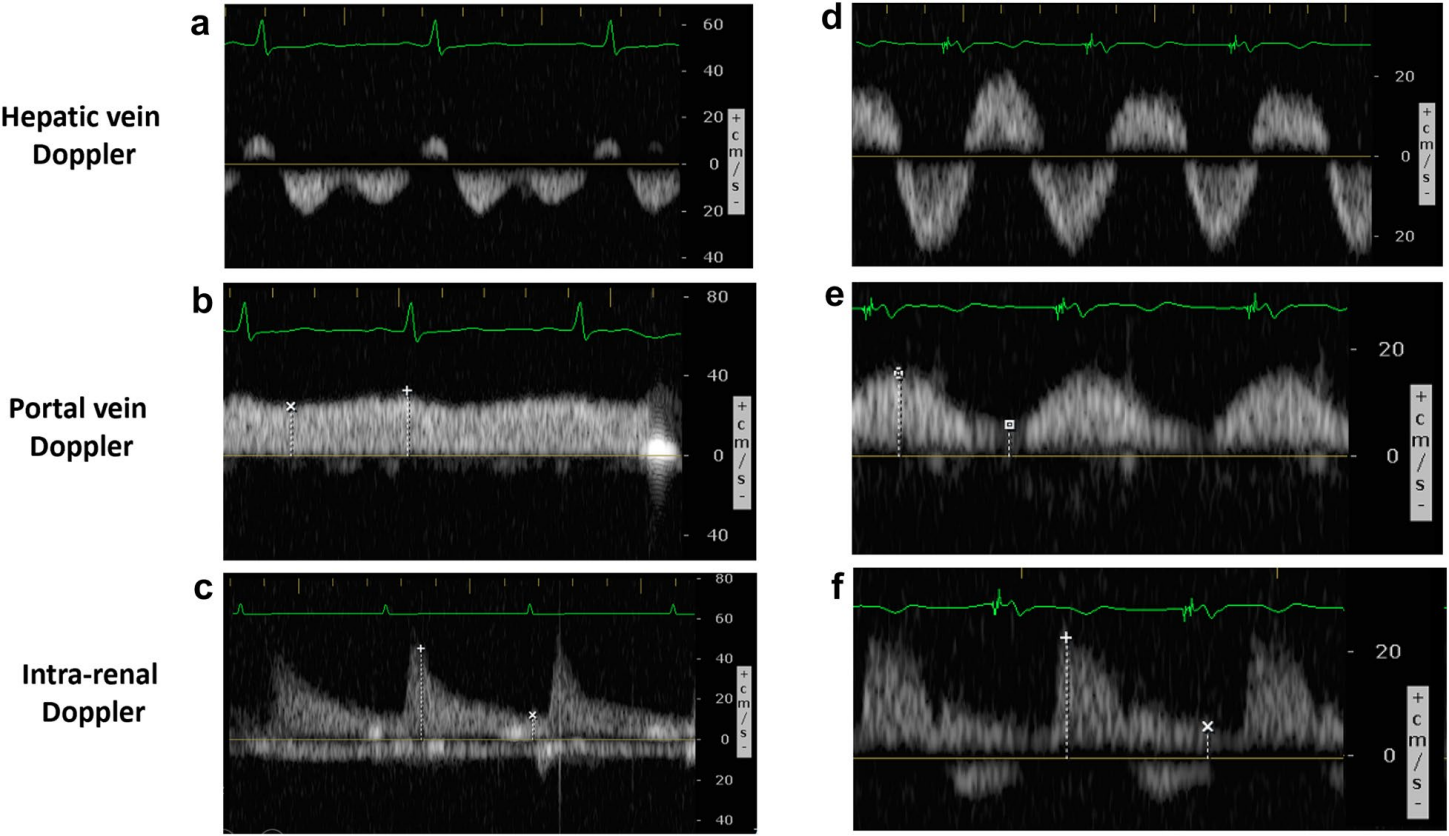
Example of VExUS C grading system assessment in cardiac surgery. Patient #1: A 55-year-old woman undergoing tricuspid valve repair aortic valve replacement and mitral valve replacement known with chronic kidney disease (baseline eGFR = 35 mL/min/1.73 m^2^) with left ventricular ejection fraction of 40% and a high risk of major complications (EuroSCORE II = 16.8%) presented the following ultrasound findings at ICU admission after surgery: a Normal hepatic triphasic pattern, b a non-pulsatile portal flow and c continuous intral-renal venous flow and an IVC diameter of > 2.1 cm (not shown) corresponding to Grade 1 of VExUS C grading system. The patient did not develop acute kidney injury, was extubated 2.2 h after ICU admission and was discharged from the ICU less than 24 h after surgery. Patient #2: A 70-year-old man undergoing mitral valve repair with a left ventricular ejection fraction of 50% and a moderate risk of major complication (EuroSCORE II = 1.54%) presented the following ultrasound findings at ICU admission after surgery complicated by right ventricular dysfunction after cardiopulmonary bypass: d Systolic reversal of the hepatic venous flow, e severe portal flow pulsatility and f severe alteration in intra-renal venous flow corresponding to Grade 3 of the VExUS C grading system. The patient developed severe acute kidney injury and delirium in the post-operative period
